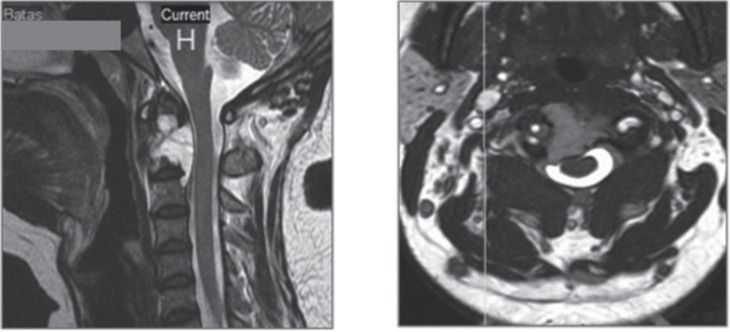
Pre-operative sagittal and axial views demonstrating the extent of the tumour on the cervical MRI.
